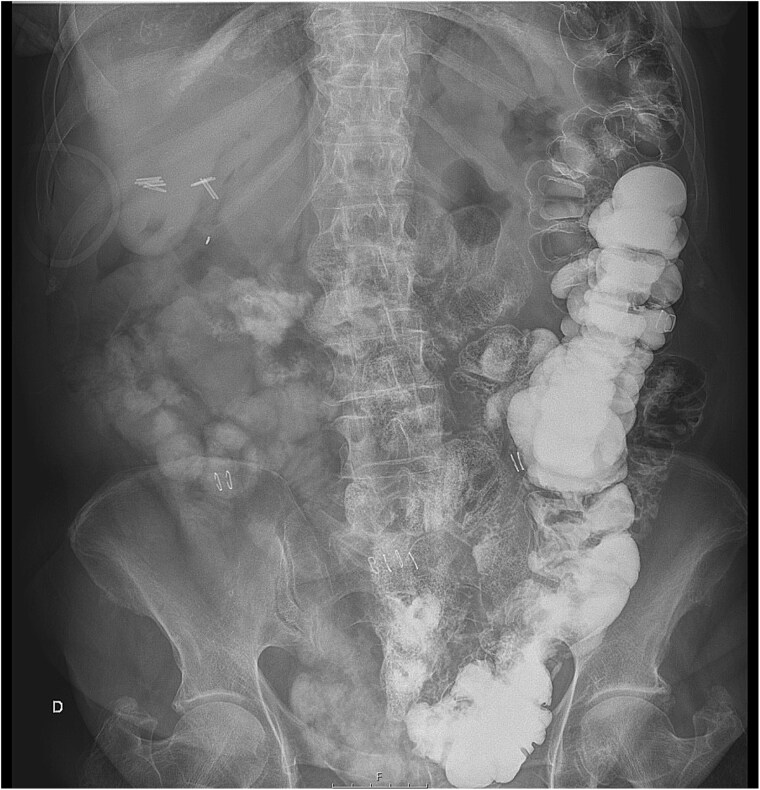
Abdominal radiography on the third postoperative day describing oral contrast on the colon which is entirely on the left side of the abdomen.
